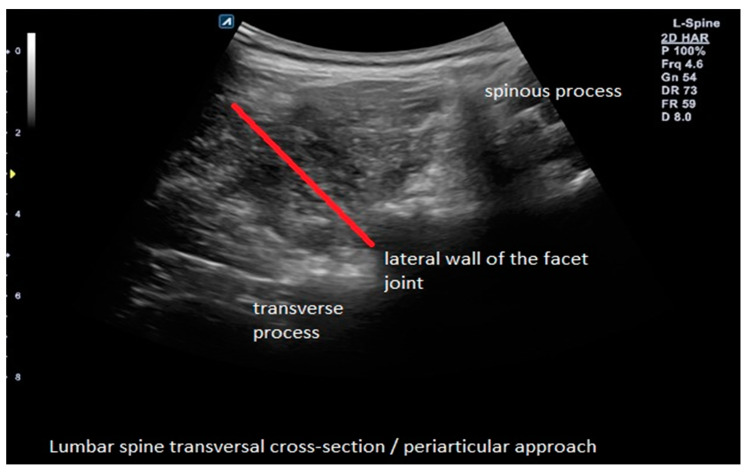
Example of ultrasound-guided perineural (periarticular) ACS injection with needle trajectory in the experimental group (B).
